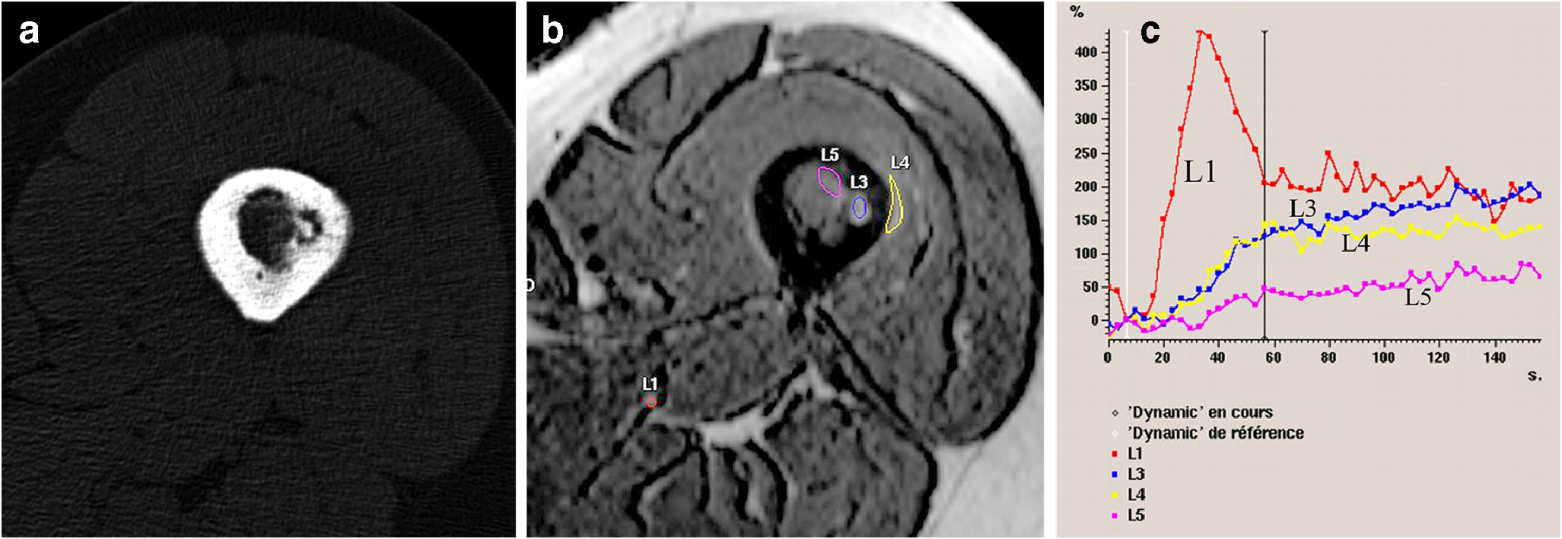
A femoral Brodie abscess on axial CT image (a) mimics an OO. However, DCE-MRI image and enhancement curves (b and c) show slow and limited enhancement in the abscess (L3) and adjacent soft tissue (L4) in comparison with a reference artery (L1). Signal enhancement in the abscess begins later than that in the artery in comparison with that expected in an OO
